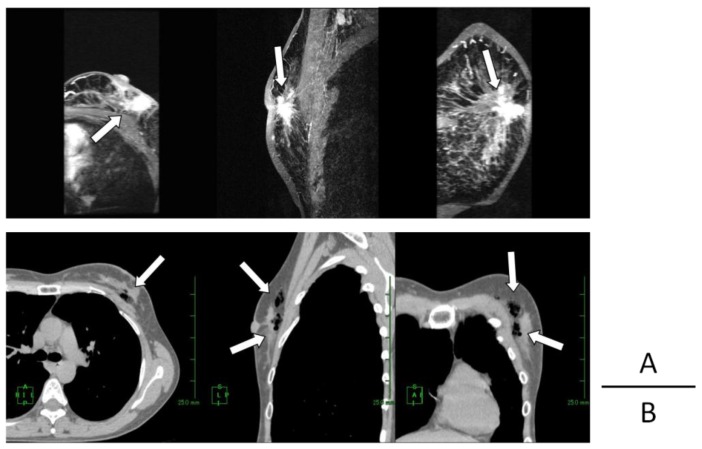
Case 2: Comparison of the position of tumor (arrows in A) and that of generated oxygen gas (arrows in B) (A) 3D-MIP (maximam intensity projection) images of pre-treatment contrast-enhanced (CE) breast MRI (B) 2D reconstructed breast CT images taken approximately 3 h after KORTUC radiosensitization agent injection.
